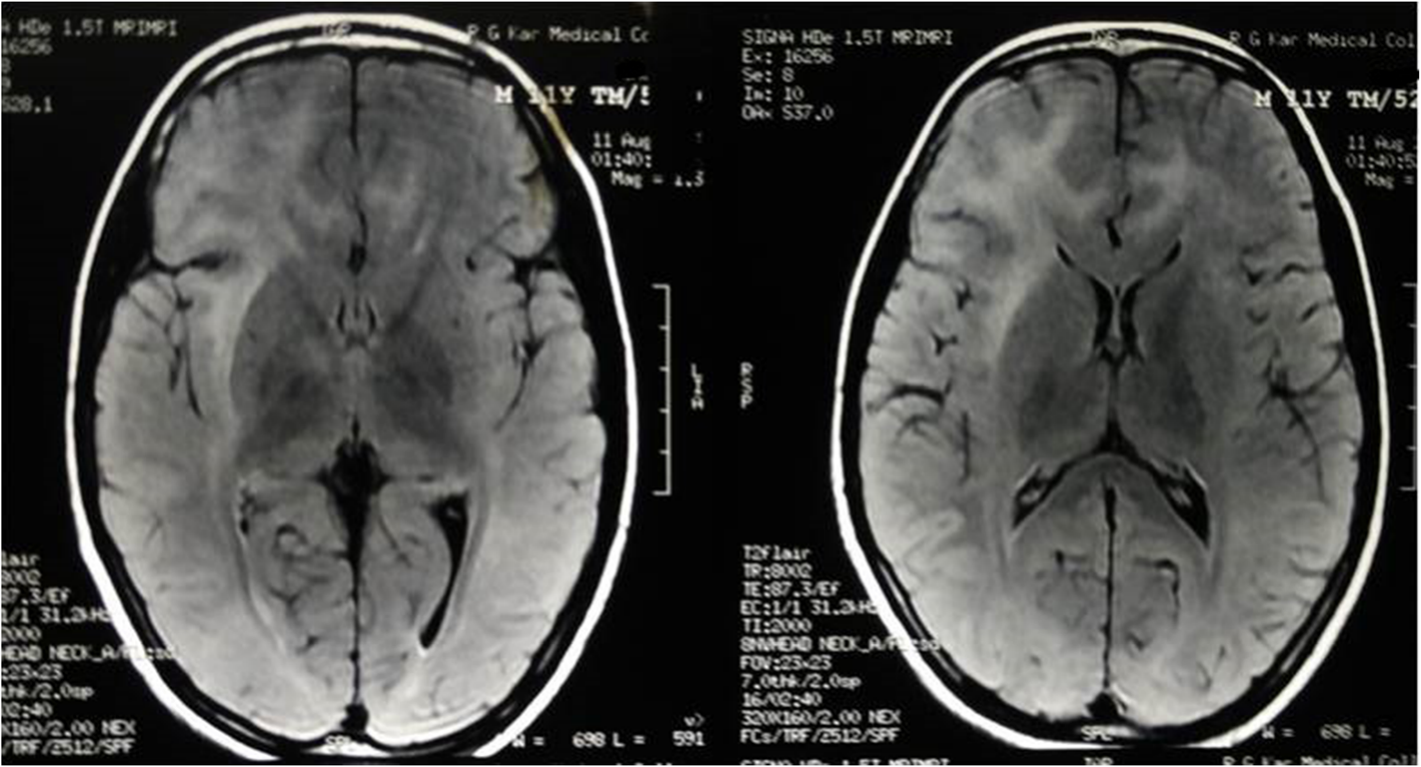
Magnetic resonance imaging of the brain (T2 fluid-attenuated inversion recovery sequence) shows white matter hyperintensities in both frontoparietal regions, more on the right side
